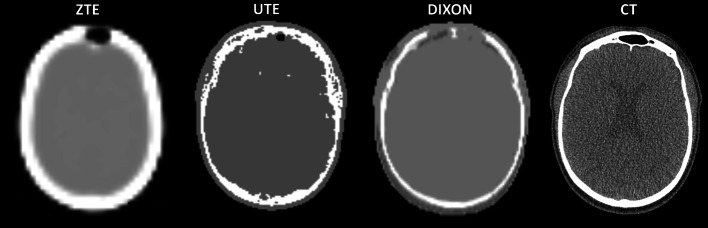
Various vendor-provided MR attenuation correction methods along with a low-dose CT used for CT attenuation correction
